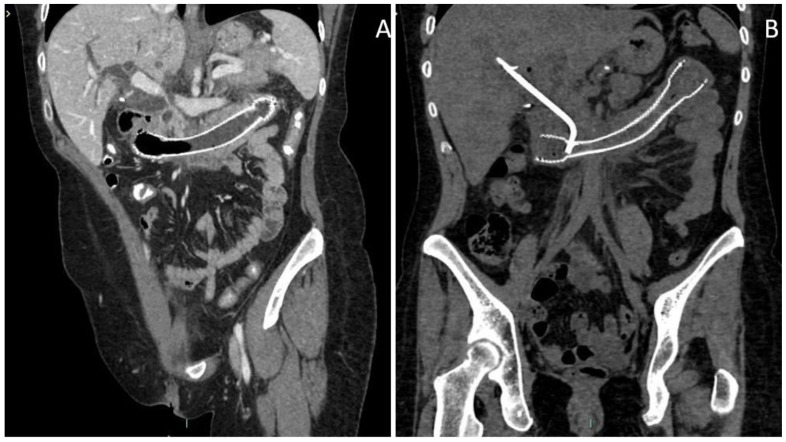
(A) Oblique MPR (Multi Planar Reconstruction) CT (Computed Tomography) image after duodenal stent placement, showing a severe dilation of the biliary tree, not present in the previous MRI exam, involving the common biliary duct. The course of the common biliary tract was grossly interrupted at the terminal end by compression of the papillary area due to the neoplastic infiltration of the stent mesh. (B) Oblique MPR CT image after biliary stent placement with the rendezvous approach due to the impossibility of reaching the papilla endoscopically due to tumor growth through duodenal stent’s mesh. Resolution of biliary dilatation is documented.
